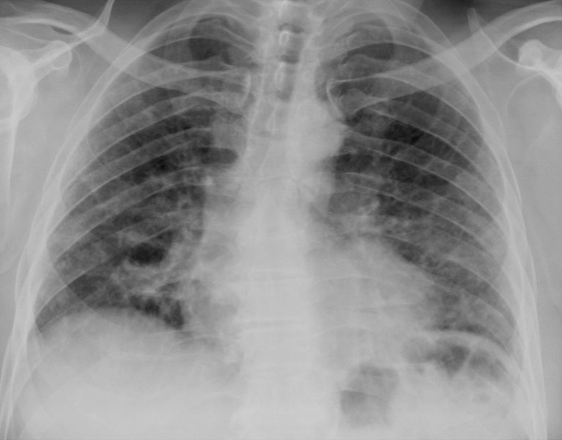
Chest X-ray in a 57-year-old man with UIP. Imaging findings are not specific and reticular pattern in the early stage of disease is not appreciable
